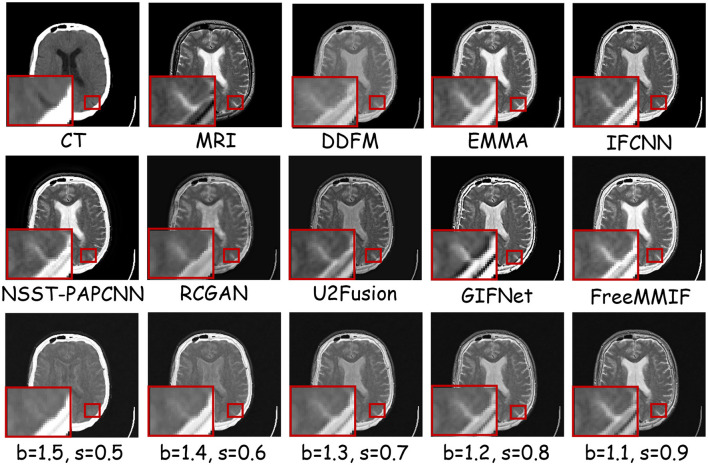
Visual comparison of CT-MRI fusion results on the Harvard Medical Dataset.
